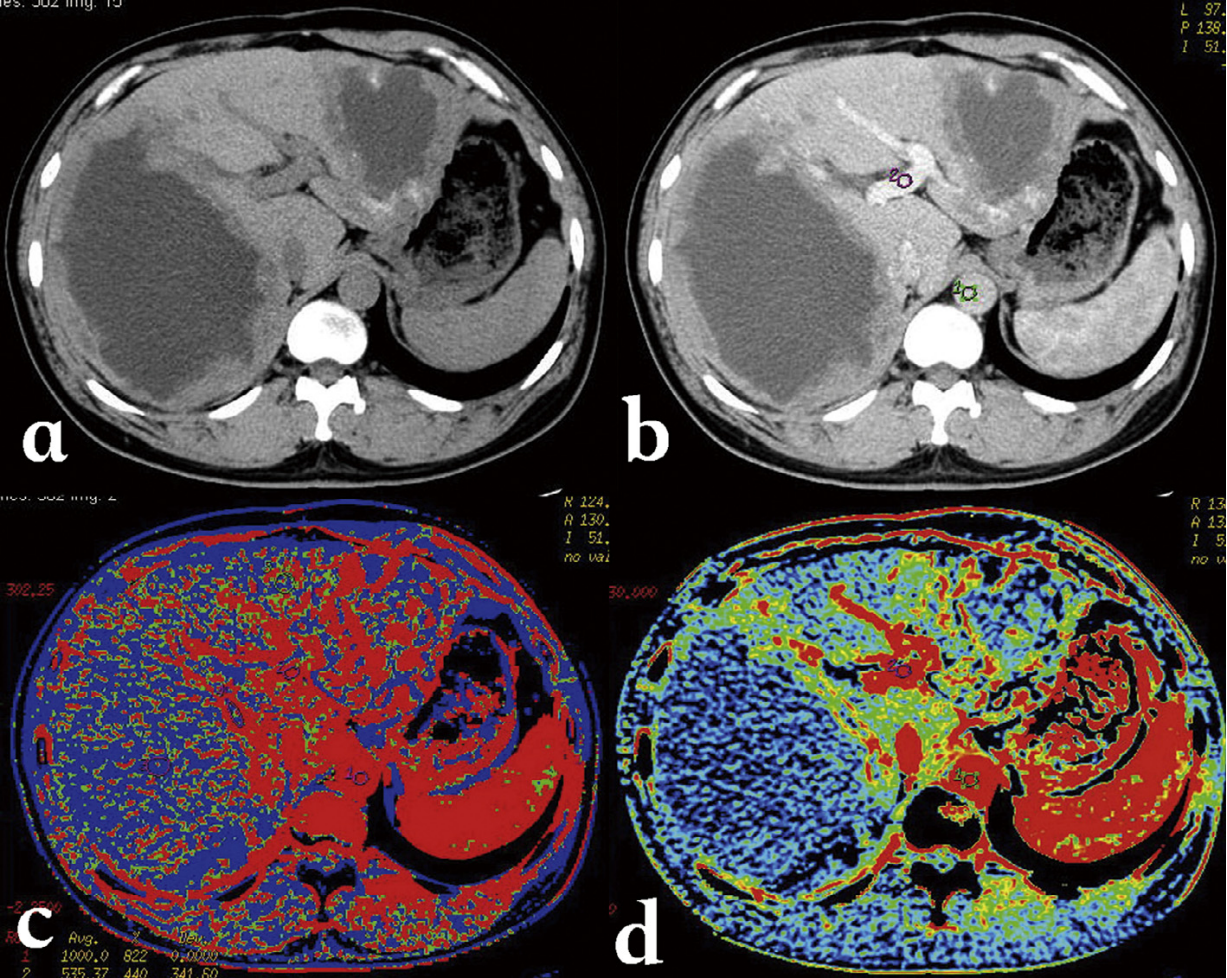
Alveolar echinococcosis in a 34-year-old man.* (a) CT plain scan shows a cyst-solid mixed hepatic mass involving both lobes of liver with irregular margin and necrosis areas of low density in the center. (b) Post-contrast phase shows a non-enhancing, hypo-attenuating lesion. (c) Blood flow map, and (d) blood volume map, at CT perfusion, show the micro-circulation in the marginal area of AE.
